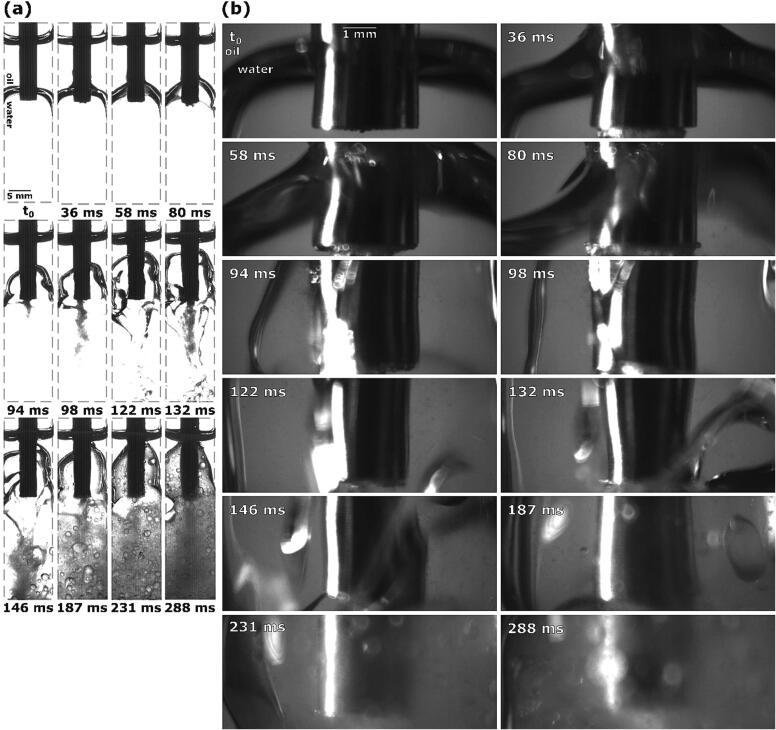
Sequence of events during ultrasonic emulsification under visible light with the ultrasonic horn positioned 2.0 mm below the oil–water interface: (a) observation with Photron Mini UX100, (b) observations with Photron SA-Z. Individual phenomena are similar to those presented on Fig. 12, mostly differing in time of appearance and appearing later due to a closer distance between horn and interface.
